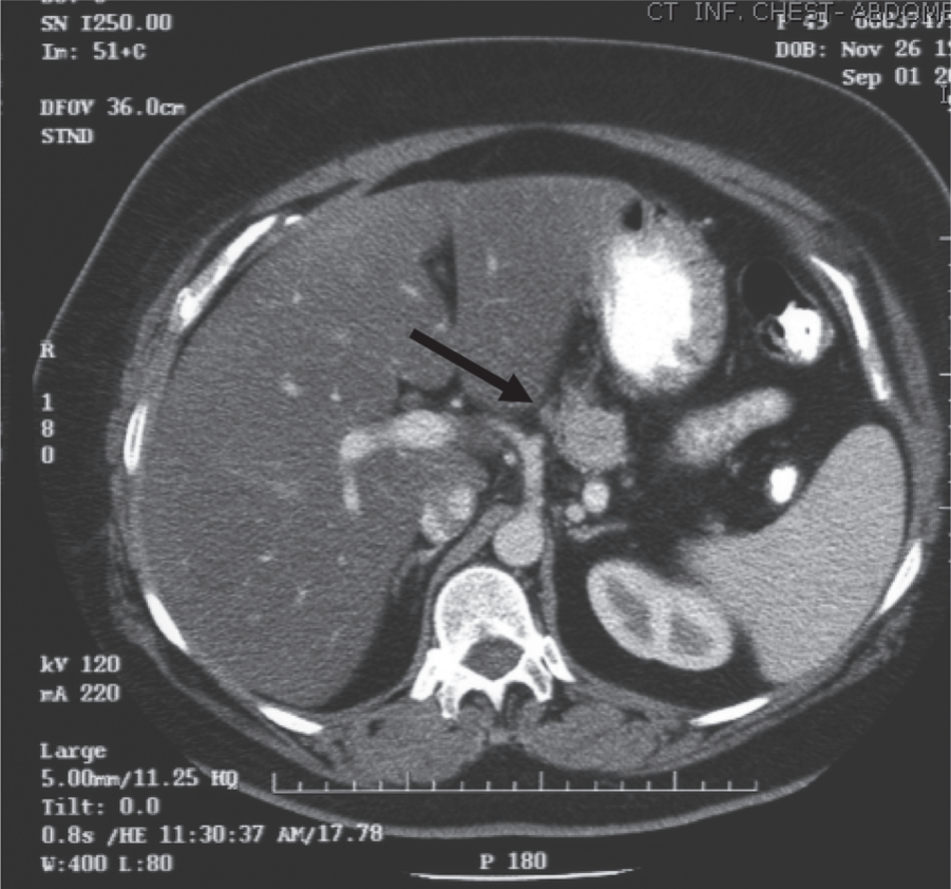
(Date: November 9, 2004) Computed tomography scan of abdomen/pelvis showed an incidental 2.5 × 2.6 cm pancreatic nonsecreting neuroendocrine tumor (arrow)
